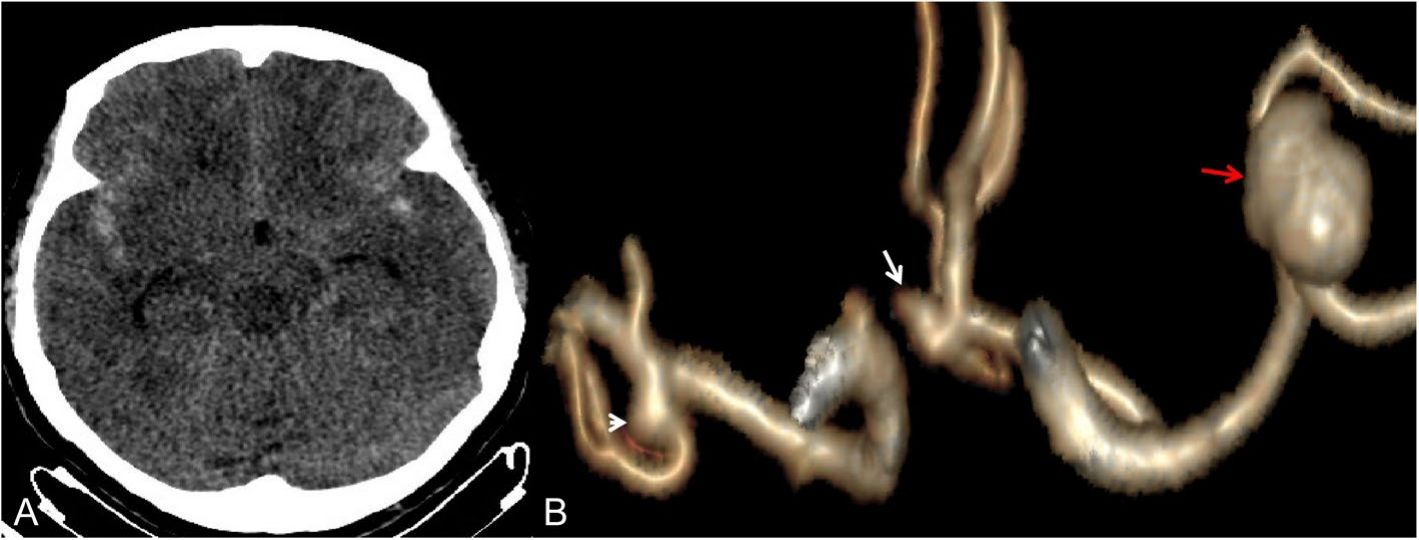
A 62-year-old female presented with symmetrical subarachnoid hemorrhage. Computed tomography angiography showed three IAs located at the left middle cerebral artery (red arrow, ruptured, aneurysm-specific prediction score = 0.59009), right middle cerebral artery (small arrow, unruptured, aneurysm-specific prediction score = -0.22189), and anterior communicating artery (lager arrow, unruptured, aneurysm-specific prediction score = 0.73085)
